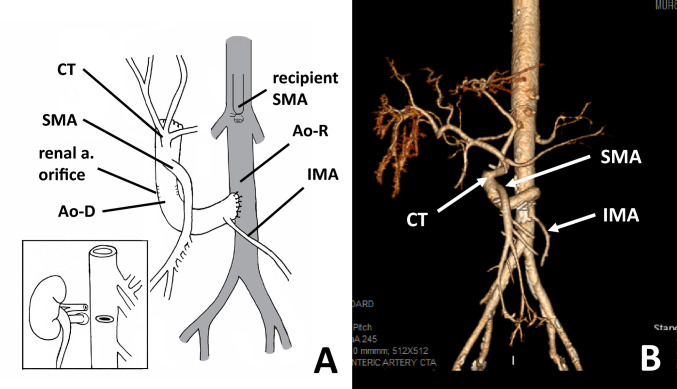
a Vascular reconstruction with preservation of all three main visceral branches, including the celiac trunk (CT), superior mesenteric artery (SMA), and inferior mesenteric artery (IMA). The renal artery orifices and the proximal aortic stump were oversewn. Vascular inflow was complete with an end-to-side anastomosis between the donor and recipient aortae (Ao-D and Ao-R, respectively). b Three-dimensional reconstruction from the CT angiogram showing preservation of all three aortic visceral branches
